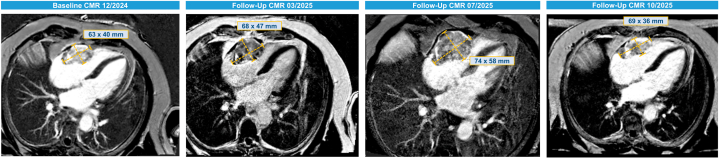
Serial CMR Follow-Up Showing Evolution of Right Ventricular Metastasis Size Serial cardiac magnetic resonance images at baseline and follow-up time points demonstrate the right ventricular mass in comparable views. CMR = cardiac magnetic resonance.
